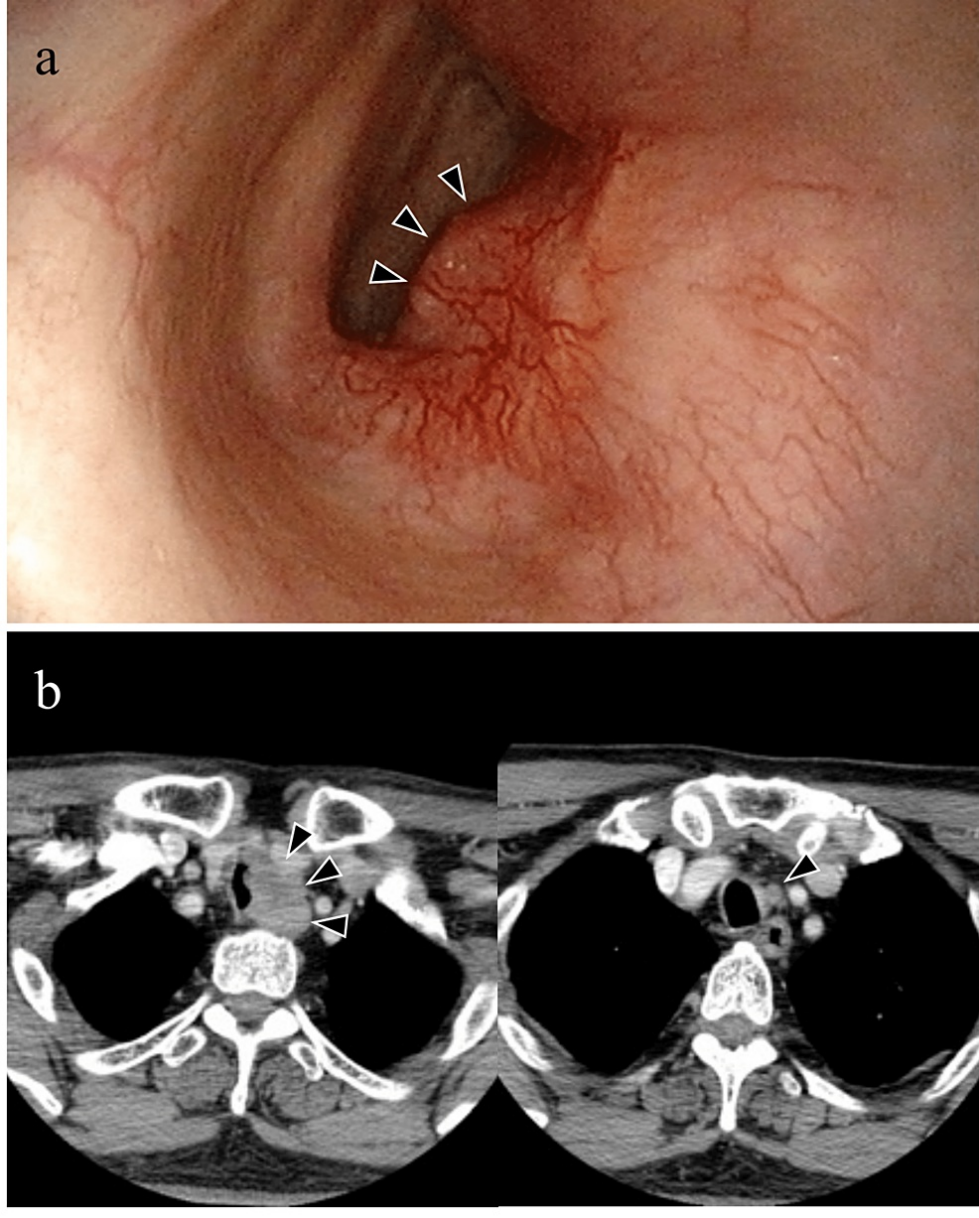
Preoperative and intraoperative findings a: Laryngeal endoscopy reveals paralysis of the left vocal cord, and arrowheads indicate a tumor protruding into the lumen of the left trachea. b: Computed tomography shows a 21 × 21 mm-sized low-concentration mass with an unclear boundary in the lower pole of the left lobe of the thyroid gland, and infiltration into the trachea was suspected. Arrowheads indicate the tumor region. Swelling of the left paratracheal lymph node was also observed.
